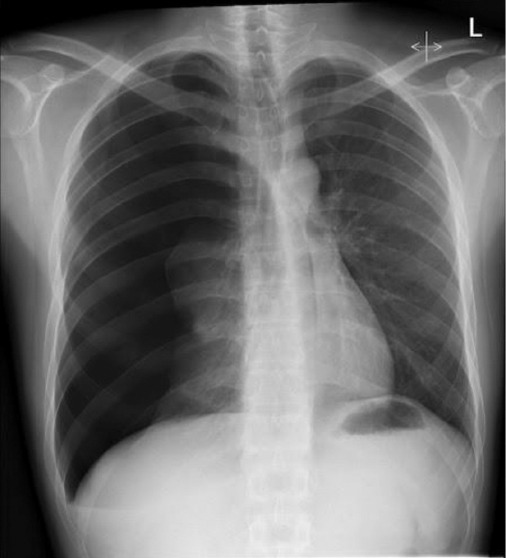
Chest radiograph of spontaneous pneumothorax
